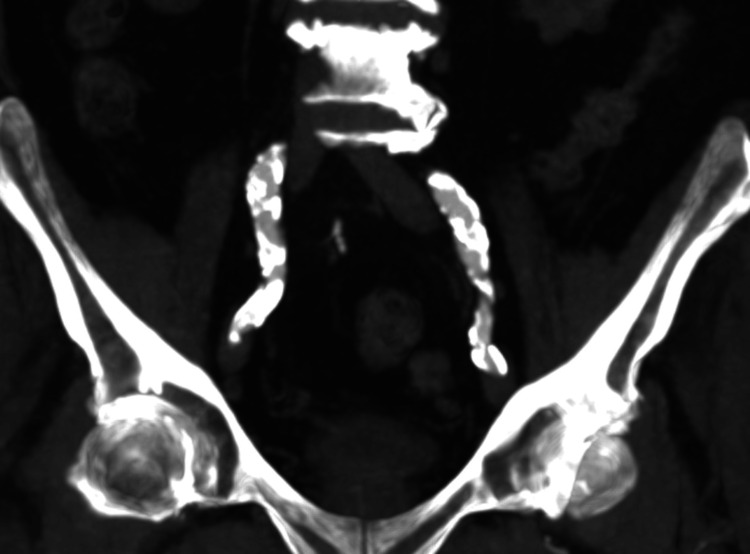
CT angiography demonstrating bilaterally heavily calcified EIAs. CT, computed tomography; EIAs, external iliac arteries
